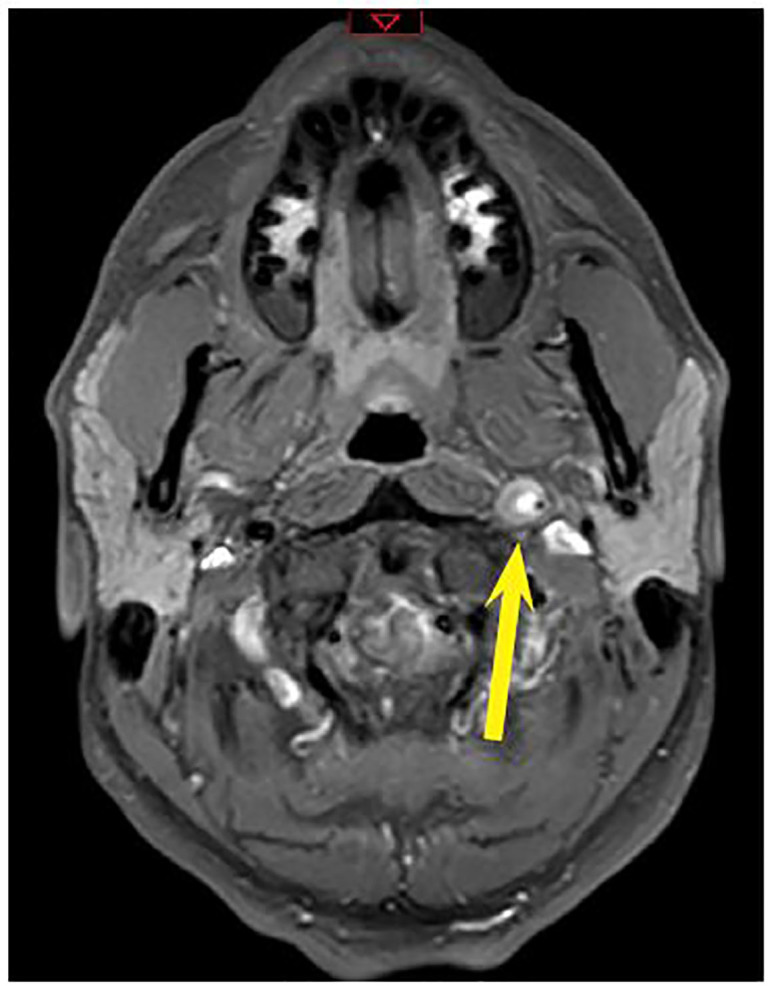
Magnetic resonance imaging (MRI) angiography T1 fat-saturated transverse view showing left internal carotid artery with significantly diminished lumen size and showing enhancement of thrombus (yellow arrow).
